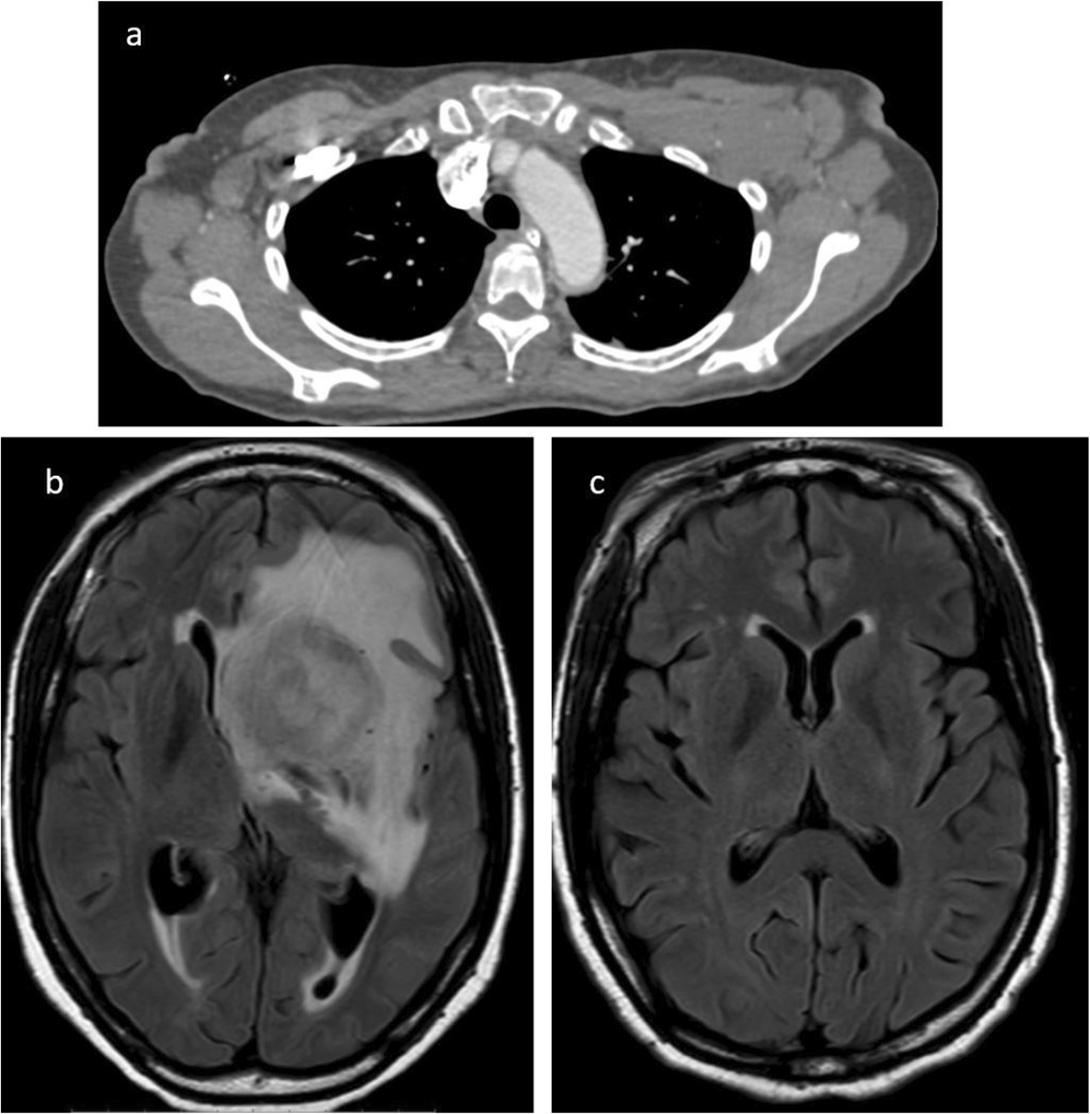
Axial CT image (a) demonstrates bilateral axillary lymphadenopathy, proven to be DLBCL after biopsy. The patient successfully completed treatment but developed CNS symptoms 1 year later. MRI of the brain (b) showed a large heterogenous mass in the left cerebral hemisphere which biopsy was proven to be lymphoma. A previous MRI brain (c) was negative for CNS involvement
